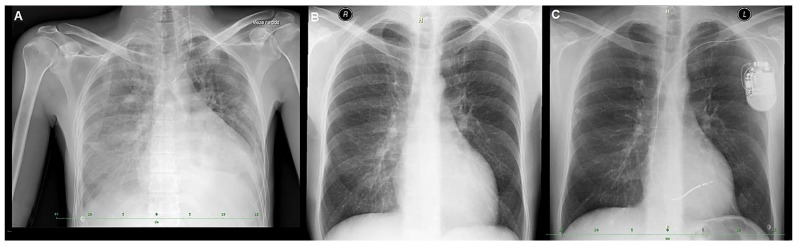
Anteroposterior chest X-ray. (A) Initial image with bilateral pleural effusion and signs of pulmonary edema. (B) Control image after pleural puncture on the left at month 1. (C) Control image after implantable cardioverter-defibrillator implantation at month 3.
